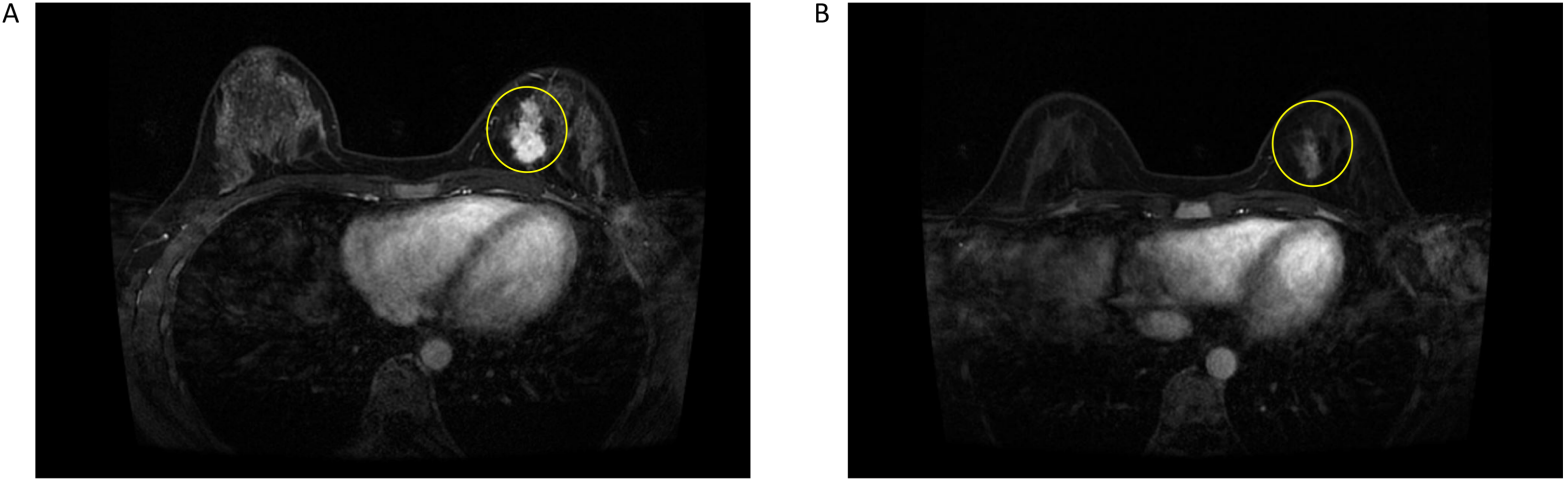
(A) Breast enhanced MRI before neoadjuvant chemotherapy. (B) Breast enhanced MRI after neoadjuvant chemotherapy.
